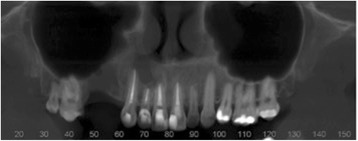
Panoramic image from computed tomography scan showing opacification in the damaged area, indicating bone repair
